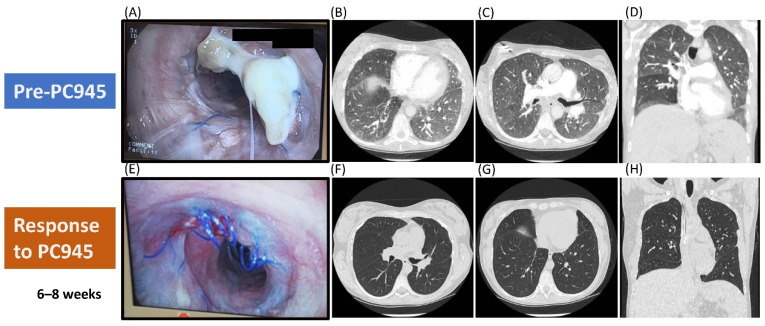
Refractory Invasive Pulmonary Aspergillosis Post Lung Transplant. Pre-exposure images (A–D) and response to Nebulised PC945 Treatment (E–H). Bronchoscopic images of the anastomosis: (A) Refractory infection pre-PC945 treatment, (E) After 8 weeks of PC945 treatment. Chest CT scans: (B–D) CT angiogram pre-PC945 treatment, (F–H) CT scan after 6 weeks of PC945 treatment. Adapted from [59] (and CT scans from Dr P Dalal, personal communication).
